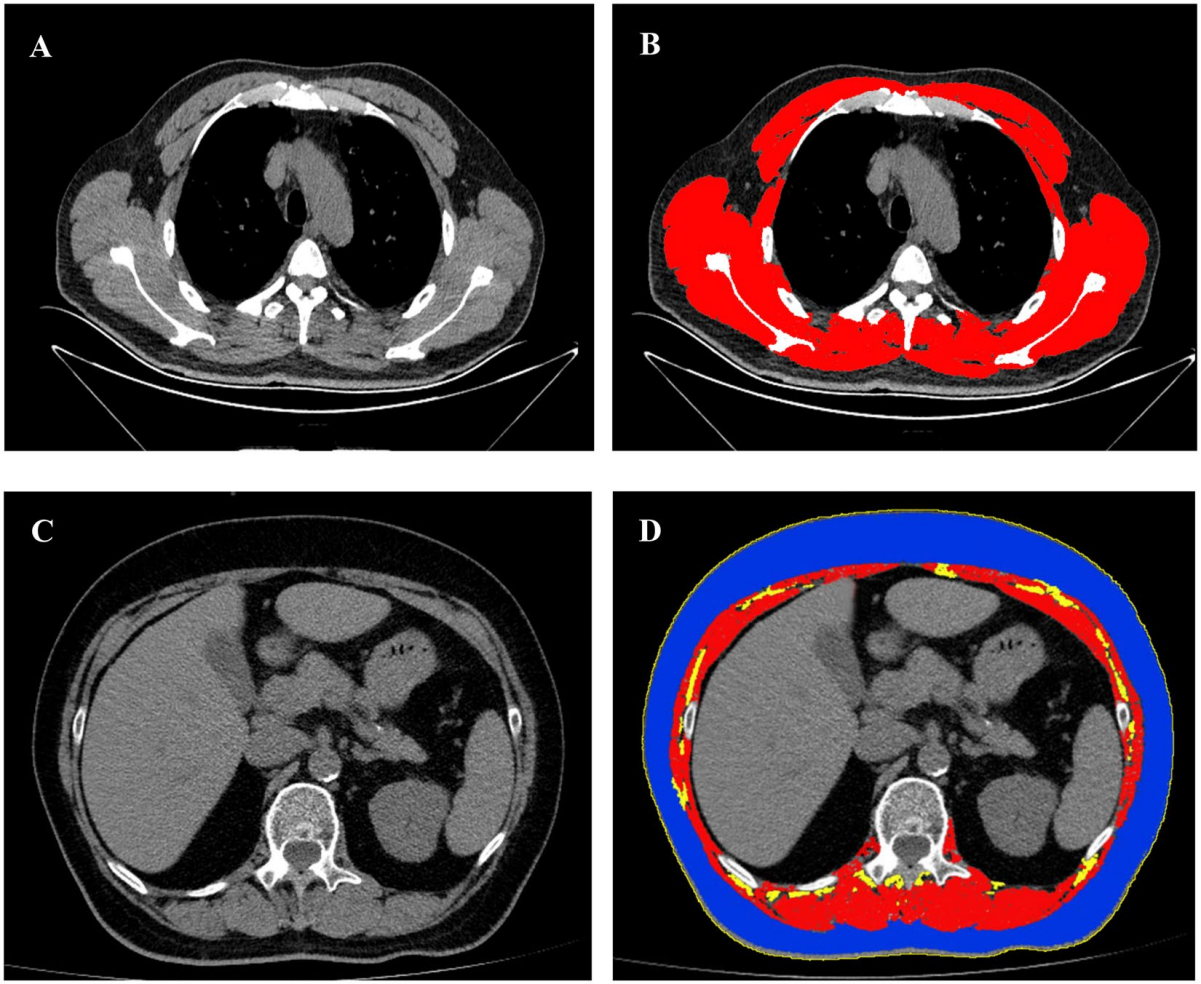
Representative examples of selected CT scan slices at the fourth thoracic vertebra level (A) with demarcated thoracic muscle (B) and at first lumbar vertebra (L1) level (C) with demarcated L1 muscle, subcutaneous adipose tissue, intramuscular adipose tissue, and abdominal circumference (D).
